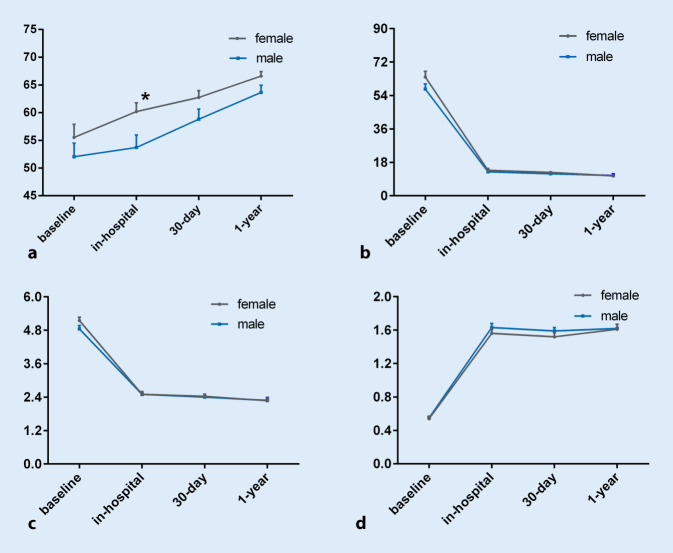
Echocardiographic data. a Left ventricular ejection fraction (LVEF, %), b mean gradient (mmHg), c maximum velocity (m/s) and d aortic valve area (cm^2^) across the aortic valve at baseline, in-hospital, 30-day and 1‑year follow-up. ^*^*p* < 0.05
